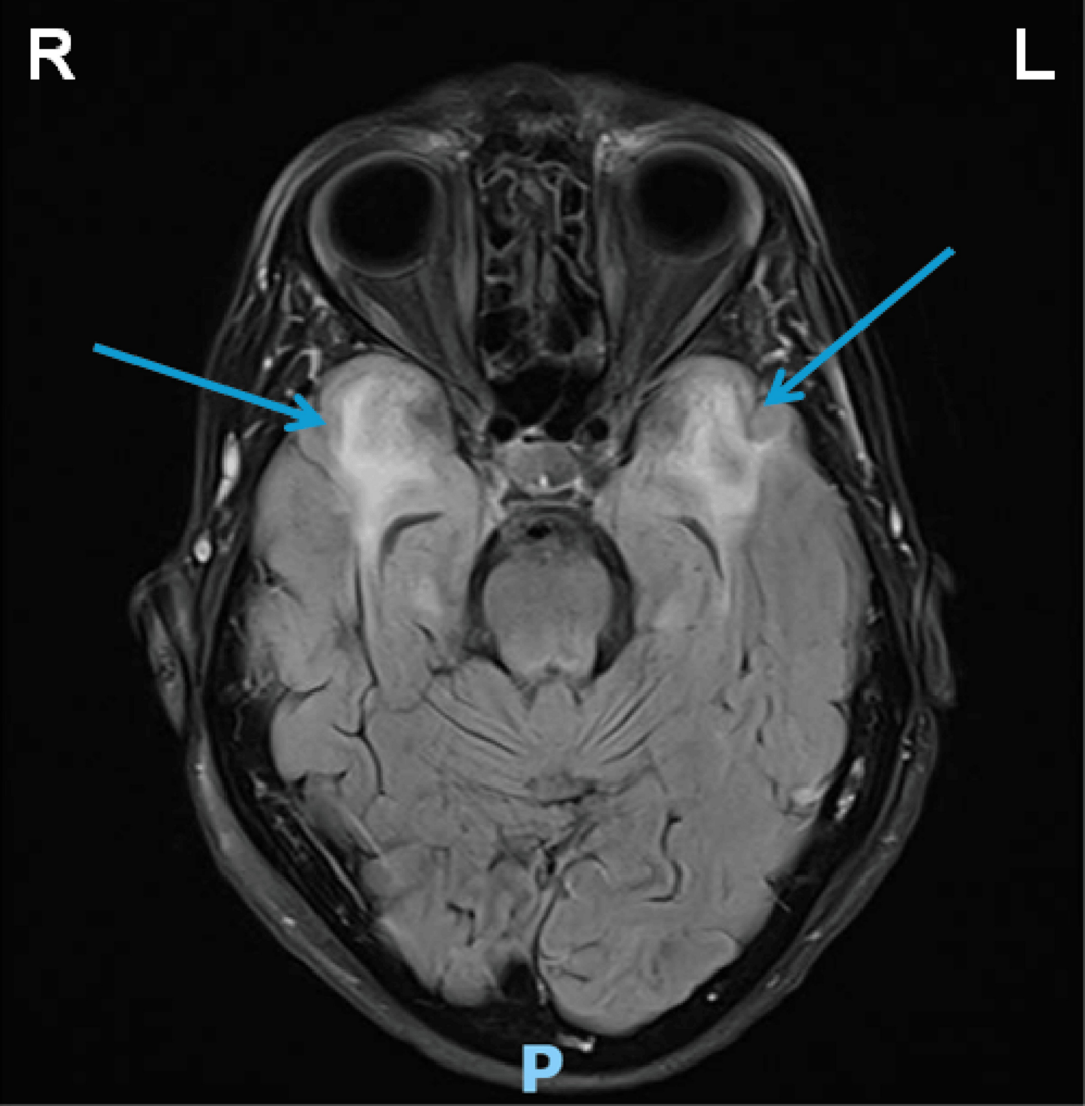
Axial MRI brain demonstrating subcortical arteriosclerotic encephalopathy (blue arrows) MRI: magnetic resonance imaging; CADASIL: cerebral autosomal dominant arteriopathy with subcortical infarcts and leukoencephalopathy Extensive high signal intensity changes in the brain parenchyma, mainly white matter. Involvement of the temporal lobes suggests CADASIL as a possible differential diagnosis
